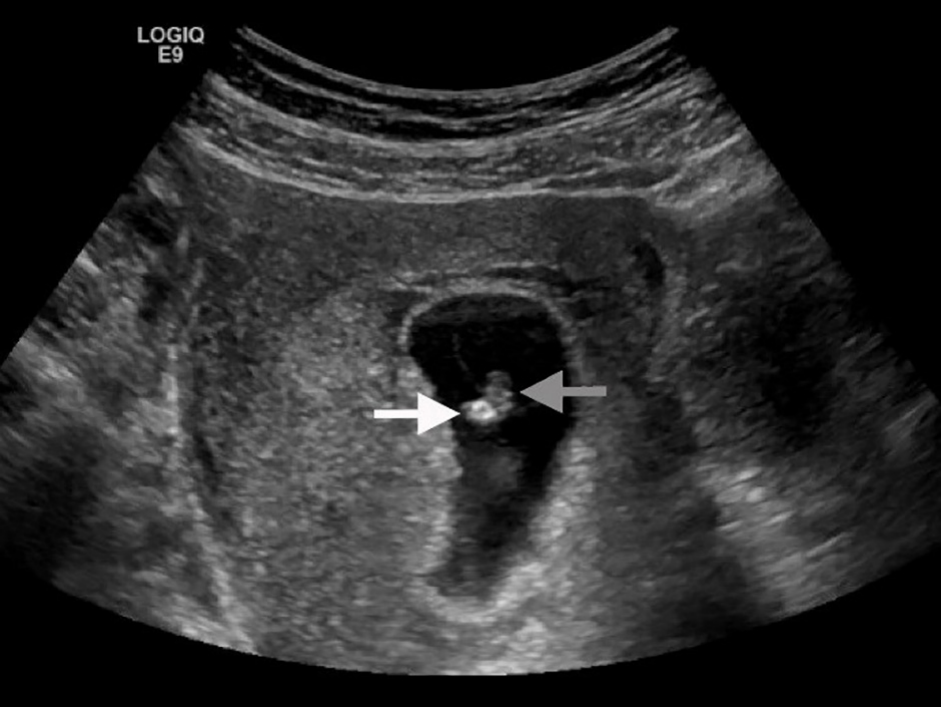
Transvaginal sonogram demonstrating a fetal pole (gray arrow) with an adjacent echogenic yolk sac (white arrow).
